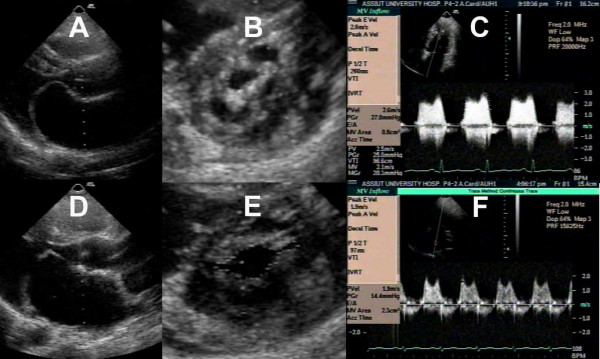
A case of mitral stenosis before and after PTMC in the parasternal long axis view (A, D), short axis view (B, E), and continuous wave Doppler transmitral flow (C, F).
